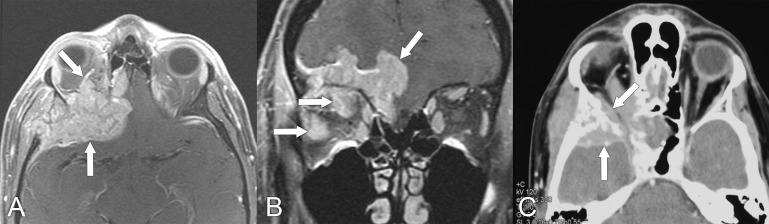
A 37-year-old man presented with right facial swelling, progressive proptosis of the right eye that developed in the last 2–3 months prior to presentation. The patient had restriction of upward gaze and complained of a pressure sensation in the right periorbital area. (A, B) Axial and coronal T1 postcontrast MRIs with fat suppression show an infiltrative mass with bony destruction of the right orbital apex and roof, with involvement of the extraocular muscles and extension into the frontal lobe (arrows). (C) CT with contrast, soft tissue window, in the same patient again shows the mass in the right orbit and middle cranial fossa with underlying bone destruction (arrows). The differential diagnosis included a metastatic lesion to the orbital apex vs. a primary bony cancer such as osteosarcoma. A biopsy was done through a craniotomy and confirmed the diagnosis of diffuse large B-cell lymphoma. This case represents an atypical presentation of lymphoma.
